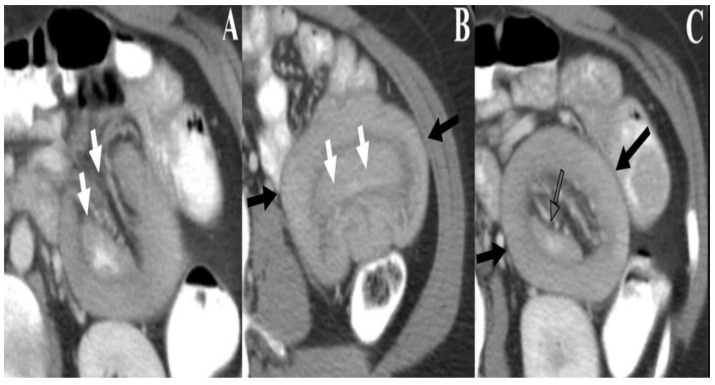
A 43-year-old patient with PJS and intestinal polyposis presented to the ER with abdominal pain. Axial contrast enhanced CT images of the abdomen (Panels A–C) demonstrate jejuno-jejunal intussusception, with telescoping of the mesenteric fat and loops of proximal jejunum (intussusceptum, white arrows) into the more distal jejunum (intussuscipiens, black arrow). An intestinal polyp was found to be the lead point and cause of intussusception (clear arrow).
